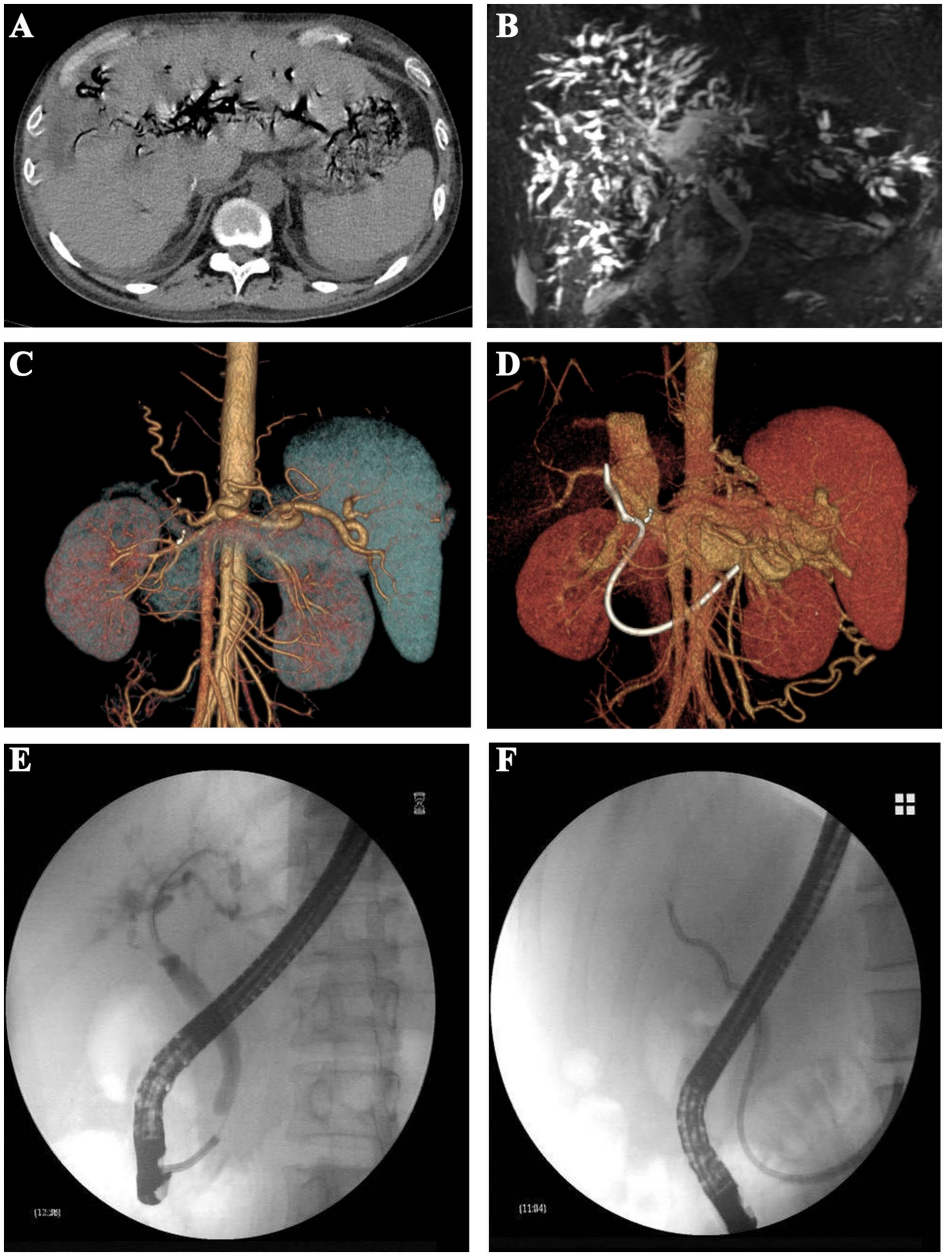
Imaging examinations and ERC procedures of the patient. (A) Abdominal contrast-enhanced computed tomography; (B) abdominal magnetic resonance cholangiopancreatography; (C) three-dimensional reconstruction of the hepatic artery; (D) three-dimensional reconstruction of the portal vein; (E) placement of biliary stents using ERC procedure; and (F) nasobiliary drainage using ERC procedure.
